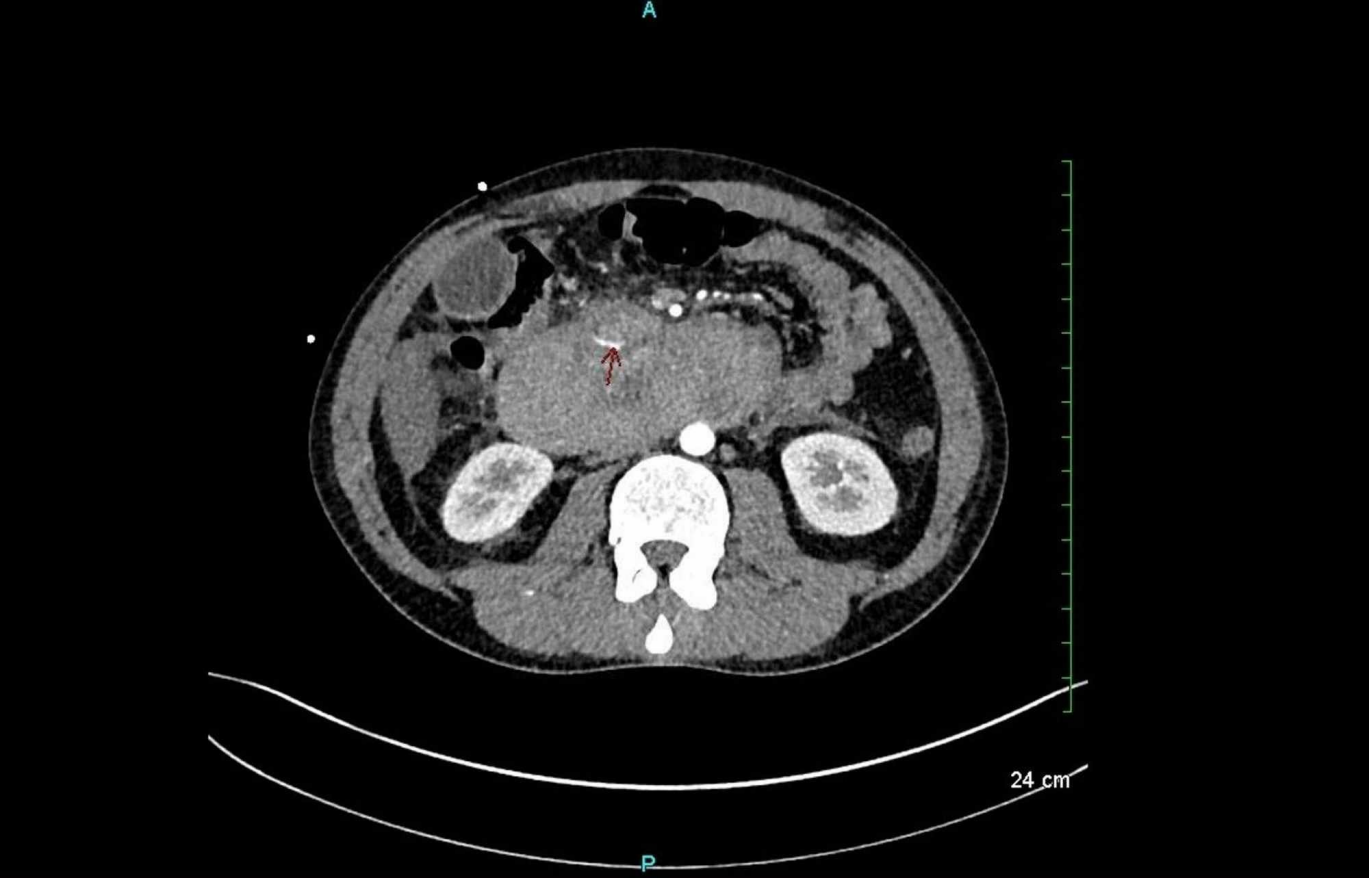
CT scan demonstrating intramural duodenal hematoma.
